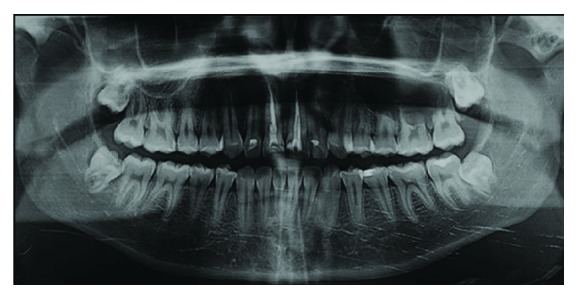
Panoramic radiograph showing in the left maxillary posterior region a radiolucent, unilocular lesion, resorptions of 2.6 roots and 2.7 mesial root, and presence of unerupted third molar.
